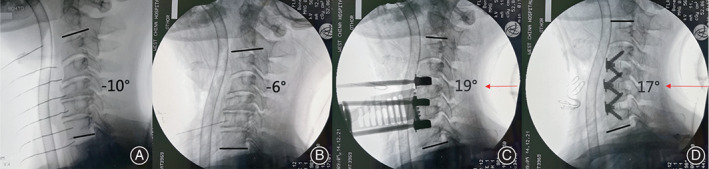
The intraoperative radiograph of a 66‐year‐old woman who underwent anterior cervical discectomy and fusion at C3‐6 level employing the intraoperative posture‐adjustment apparatus. The radiograph showed C2‐C7 lordosis (CL) was −10° at the beginning of the surgery (A). When decompression steps were accomplished, the intraoperative radiograph showed CL was −6° (B). As the pillow arising (labeled with red arrow), the CL changed to 19° (C). When Zero‐P was inserted, the CL suffered a tiny decreased a little to 17° (D).
